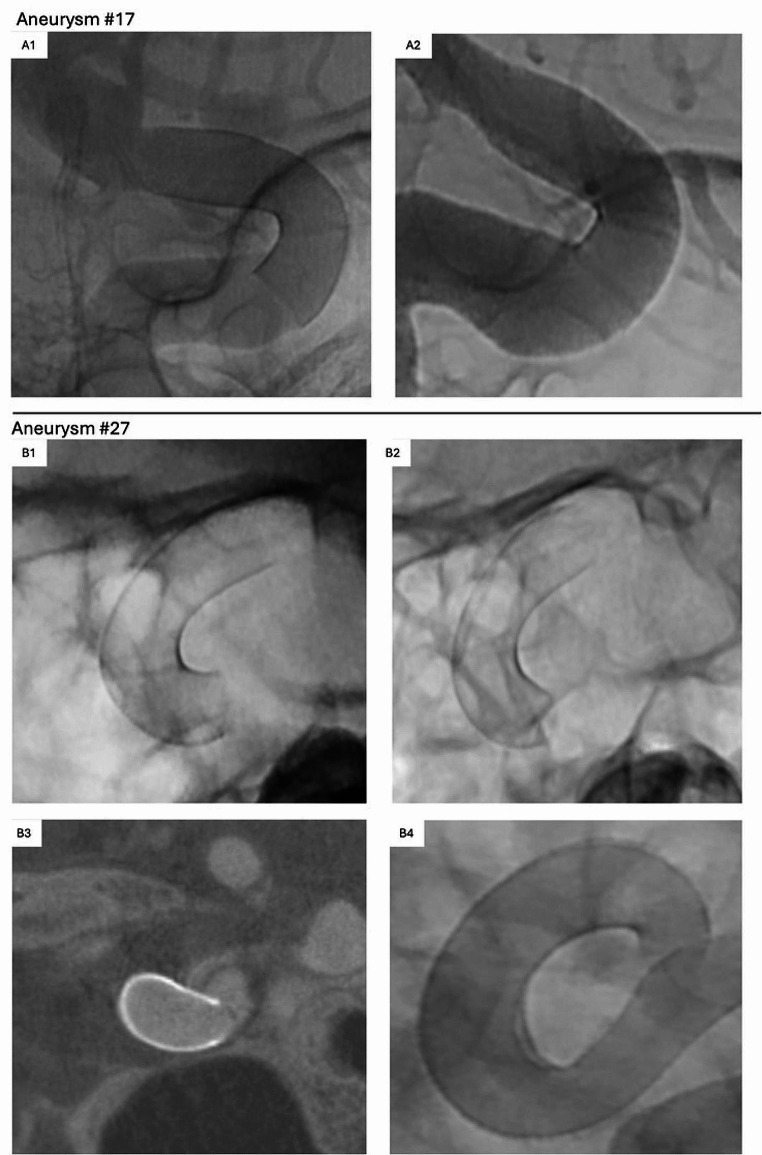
Device Visibility & Fish Mouthing Example. *(A1/2)*: Postinterventional control after FD deployment with incomplete apposition of the proximal FD to the parental vessel curvature (A1) with FD retraction and complete vessel wall adaptation based on follow-up DSA 257 days after intervention (A2). *(B1-4)* Progressive proximal fish mouthing: Initial post-deployment projection (B1), compared with a control projection the following day (B2) after initial suspicion was noticed by a CT at the first postinterventional day; followed by the decision for a second FD deployment with FPCTA before the second FD deployment (B3) and control angiogram after second FD deployment (B4). FD, flow diverter; DSA, digital subtraction angiography
